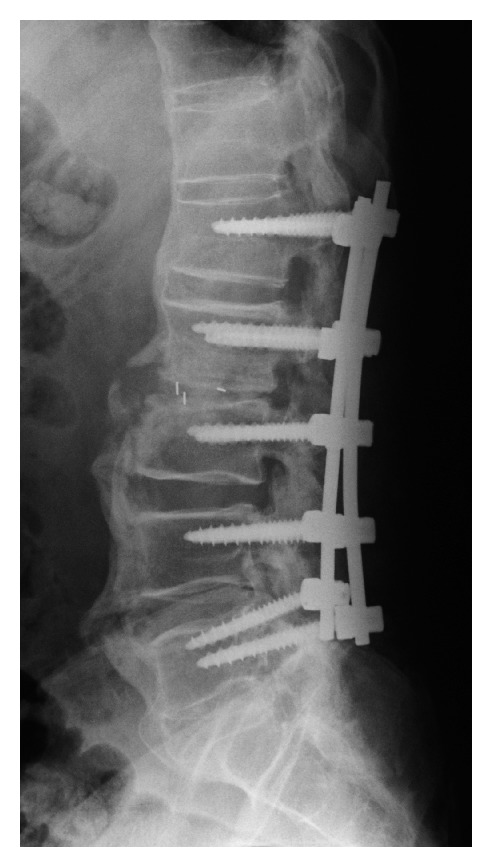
Lateral plain X-ray image obtained after the first surgery. In addition to posterior fusion at the L1–L5 intervertebral space, posterior lumbar interbody fusion at the L2-L3 intervertebral space was performed using a PEEK cage and local bone.
